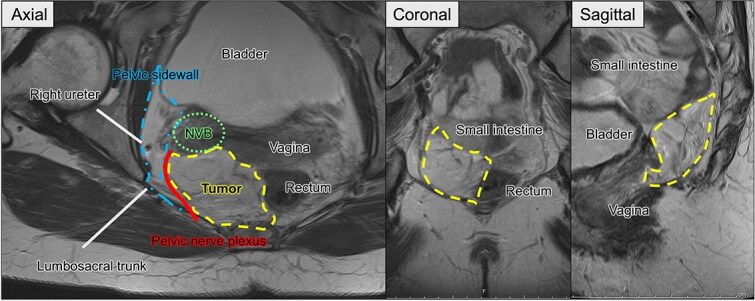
Preoperative pelvic MRI showing the extent and anatomical relationships of the recurrent tumor (dashed line). Axial view: The tumor is located adjacent to the rectum and vagina, abutting the pelvic sidewall (dash-dotted line), neurovascular bundle (NVB) (dotted line), pelvic nerve plexus (solid line), and right ureter. Coronal and sagittal views. The tumor is observed near the small intestine, rectum, bladder, and vaginal stump.
